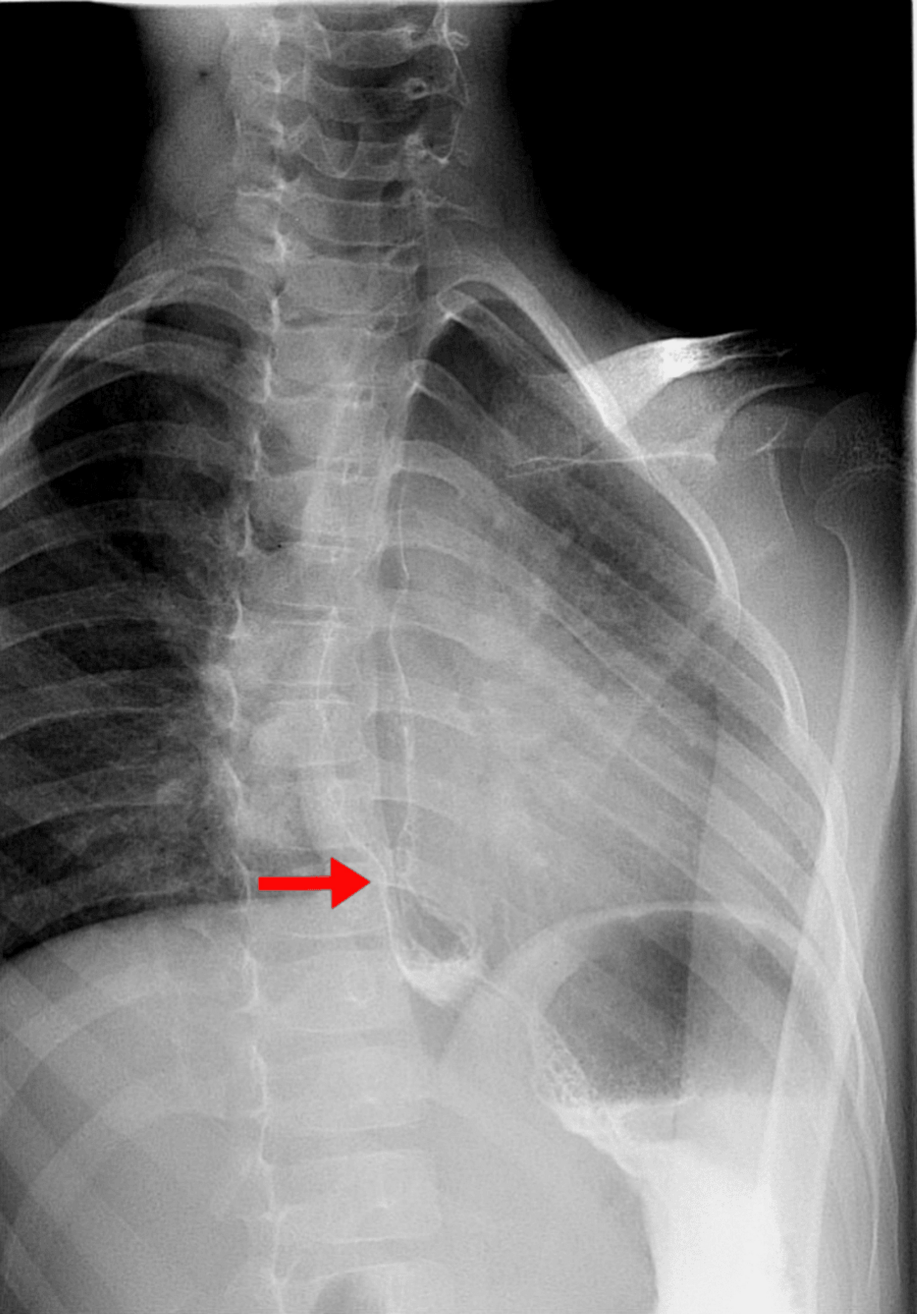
Barium swallow X-ray showing focal persistent narrowing seen within the distal aspect of the esophagus (red arrow) suggestive of fibrosis.
